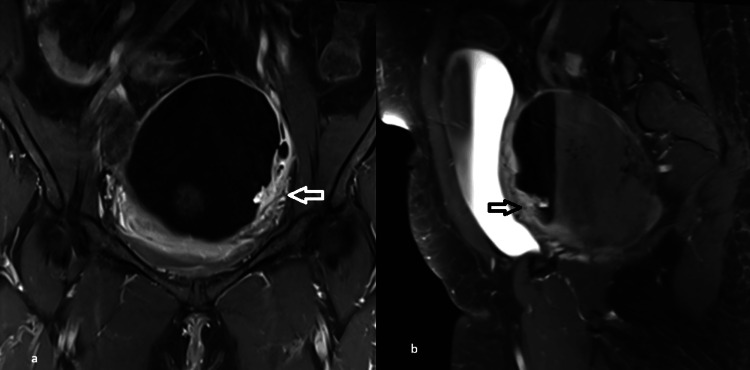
Multiplanar MRI revealing irregular enhancing mural thickening MRI: magnetic resonance imaging (a, b) Coronal and sagittal T1-weighted fat-saturated postcontrast images demonstrating irregular enhancing mural thickening along the cyst wall (arrows), better appreciated on multiplanar imaging
